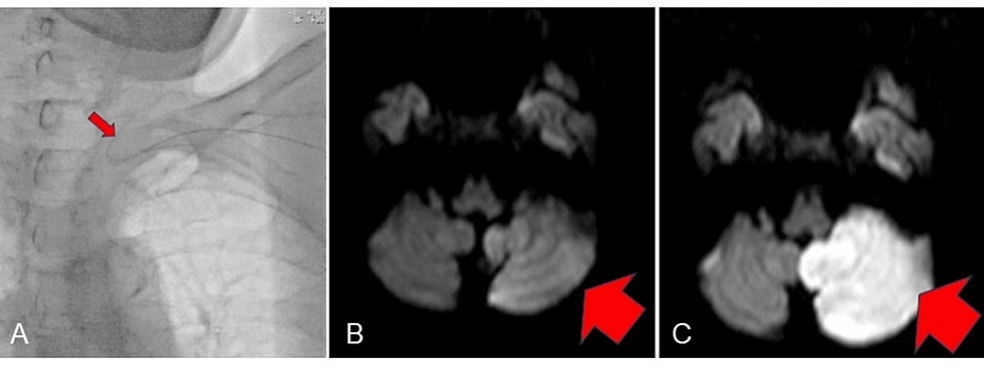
Case of left cerebellar infarction caused by RAVI (A) A guide wire was inadvertently inserted into the left vertebral artery. (B) MRI (DWI) was performed immediately after onset.  (C) MRI (DWI) was performed on the day after onset. RAVI, radial access for visceral intervention; DWI, diffusion-weighted imaging
